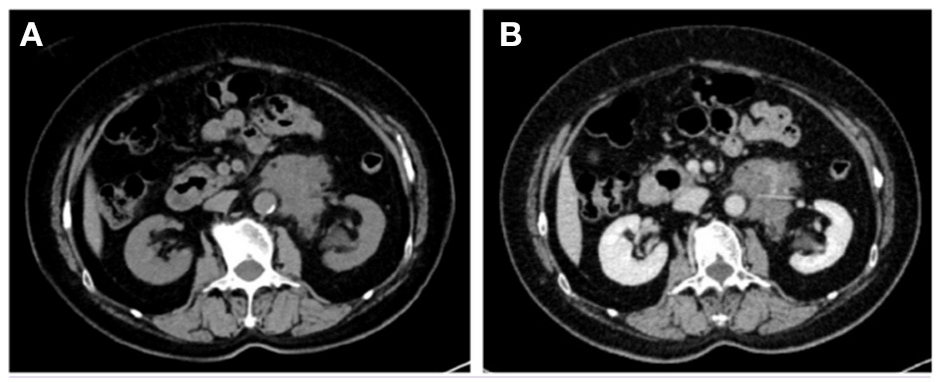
CT showed an irregular retroperitoneal mass of mixed density with coarse margin between the abdominal aorta and the left renal hilum in plain CT (A). The mass was shown as heterogeneous enhancement in the delayed phase (B).
